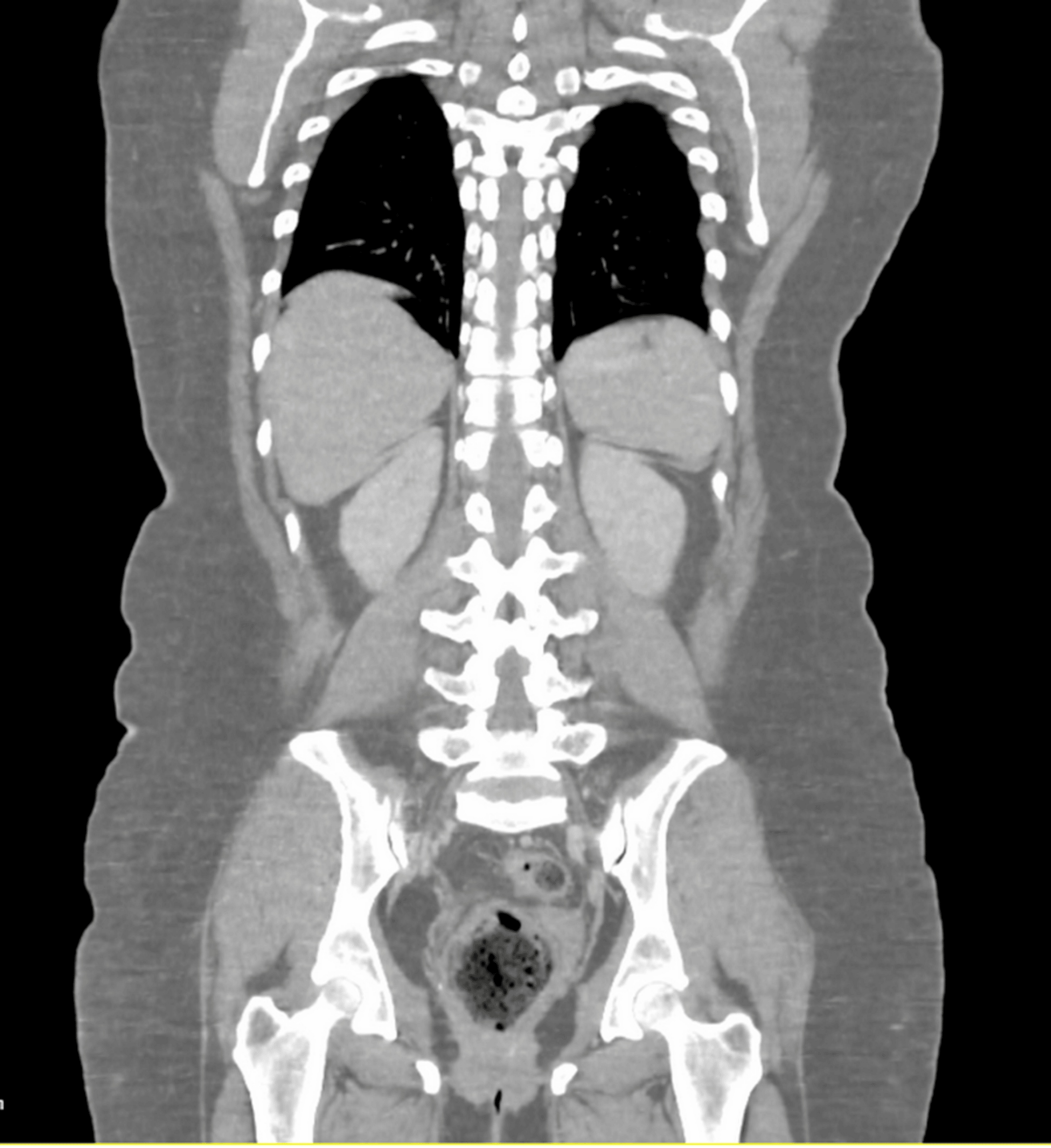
CT scan image of thorax, abdomen, and pelvis with contrast showing no significant abnormalities in bone structure.
